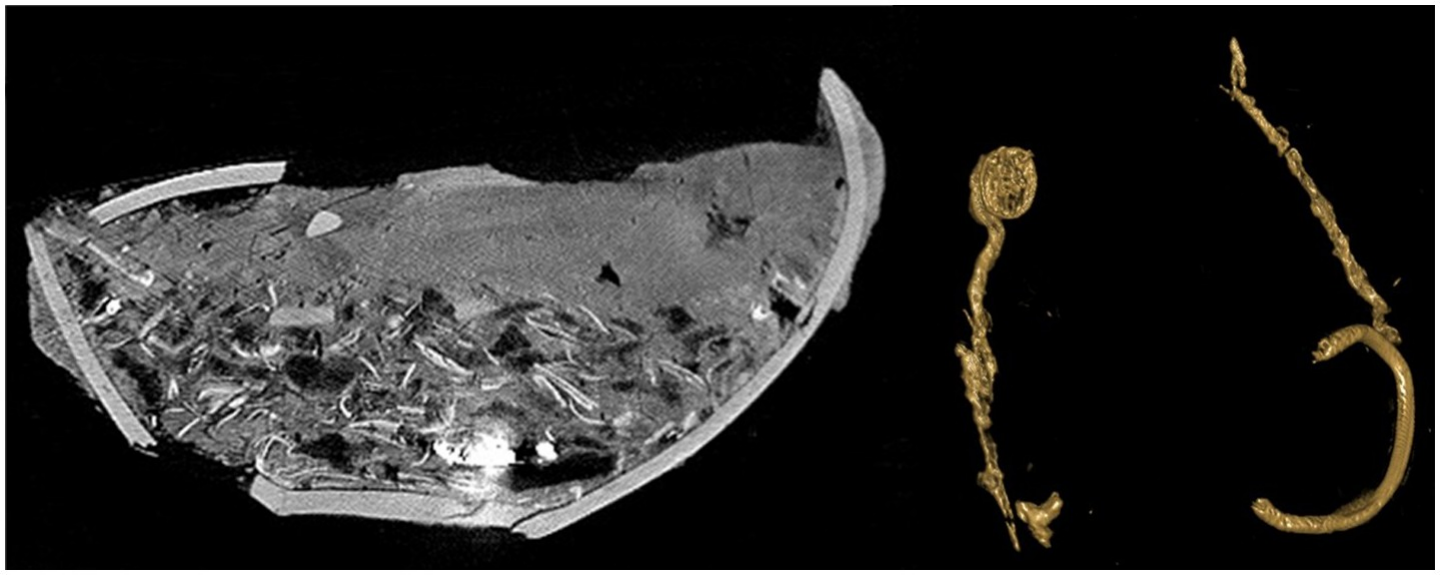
CT images of urn grave A278 from Aarre urnfield. To the left is a cross section of the fragmented urn that contain a bottom layer of cremated bone and some fragments of metal. To the right the metal has been isolated and it is possible to determine a bronze ring and two pins with disc-shaped head. Urn and artefacts are not to scale Modified by H. A. Rose and published under a CC BY license 4.0 with permission from original copyright holder Arkæologi Vestjylland).
